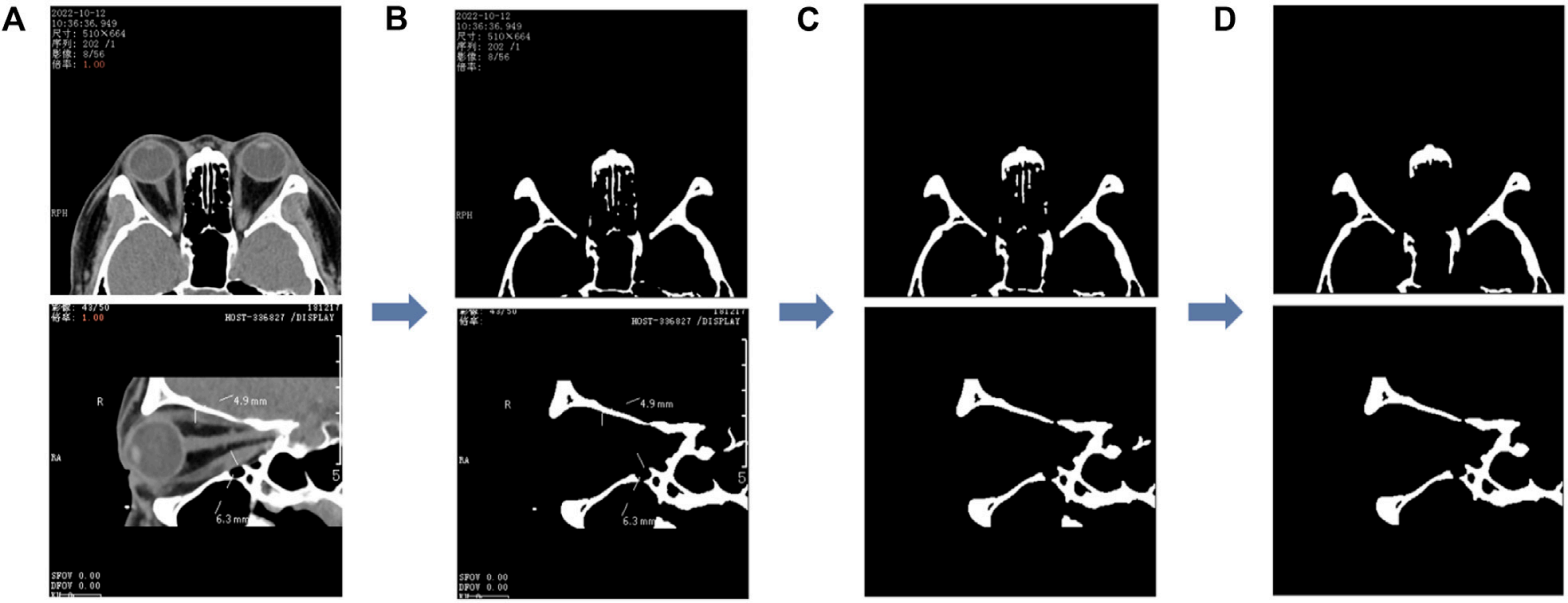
Image processing of the segmented orbital rim region. (A) The unprocessed CT image and the binary image in (B) are obtained after the threshold segmentation; the binary image shown in (C) is obtained after the morphological opening operation, and finally, the smaller connected domain is eliminated to obtain the binary image containing the outer edge of the orbit shown in (D). The first row represents the axial CT image and the second row the sagittal CT image.
